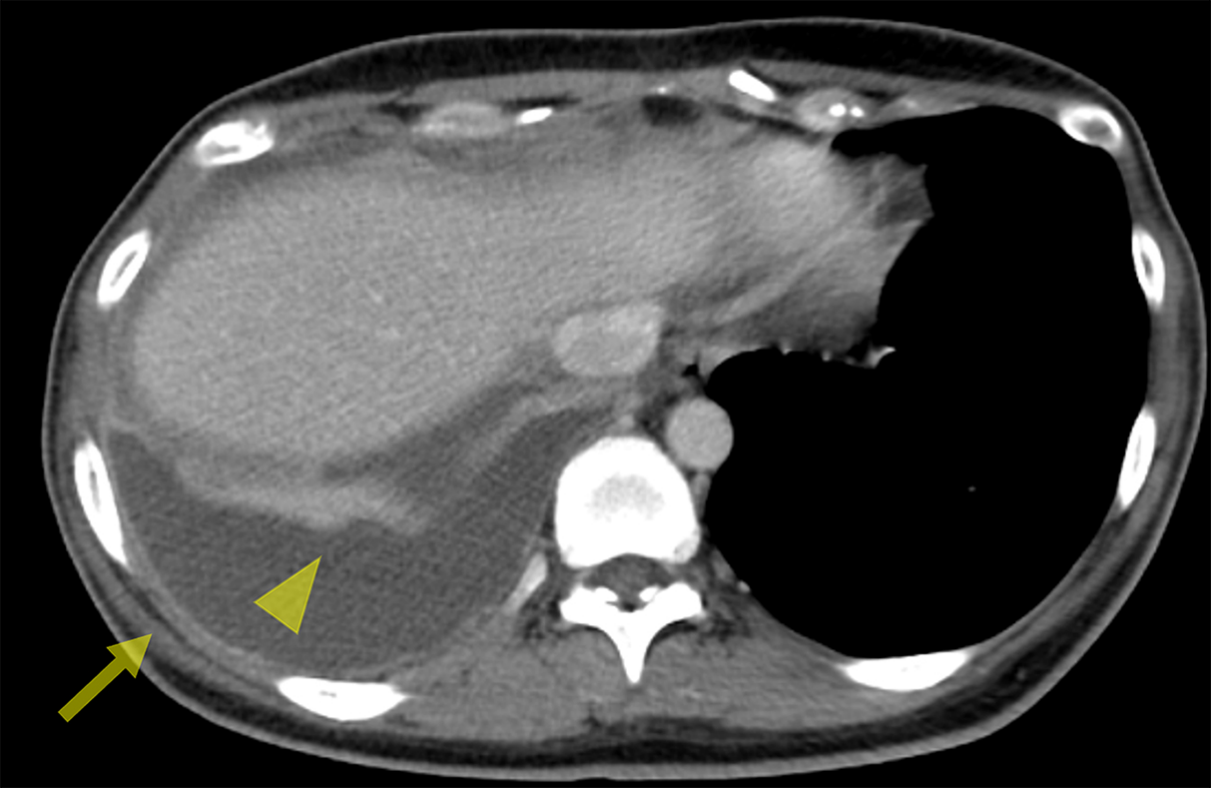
Right pleural effusion with enhanced pleural thickening (arrow) and subpleural nodules (arrowhead) in the right upper lobe on contrast-enhanced chest CT. CT: computed tomography.
